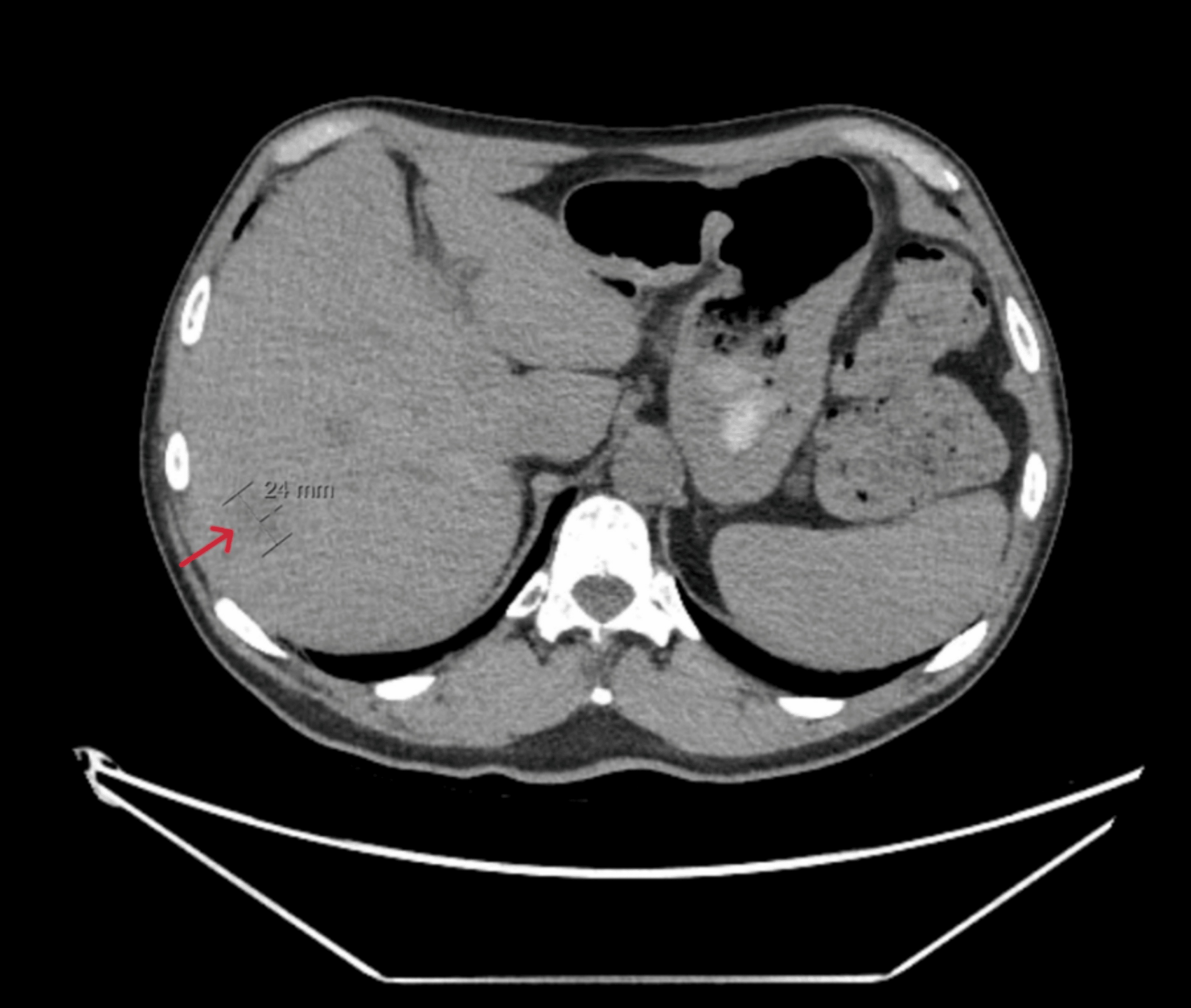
Non-contrast chest CT demonstrating new ill-defined, low-attenuation lesions throughout the liver, measuring up to 2.4 cm in the right hepatic lobe
